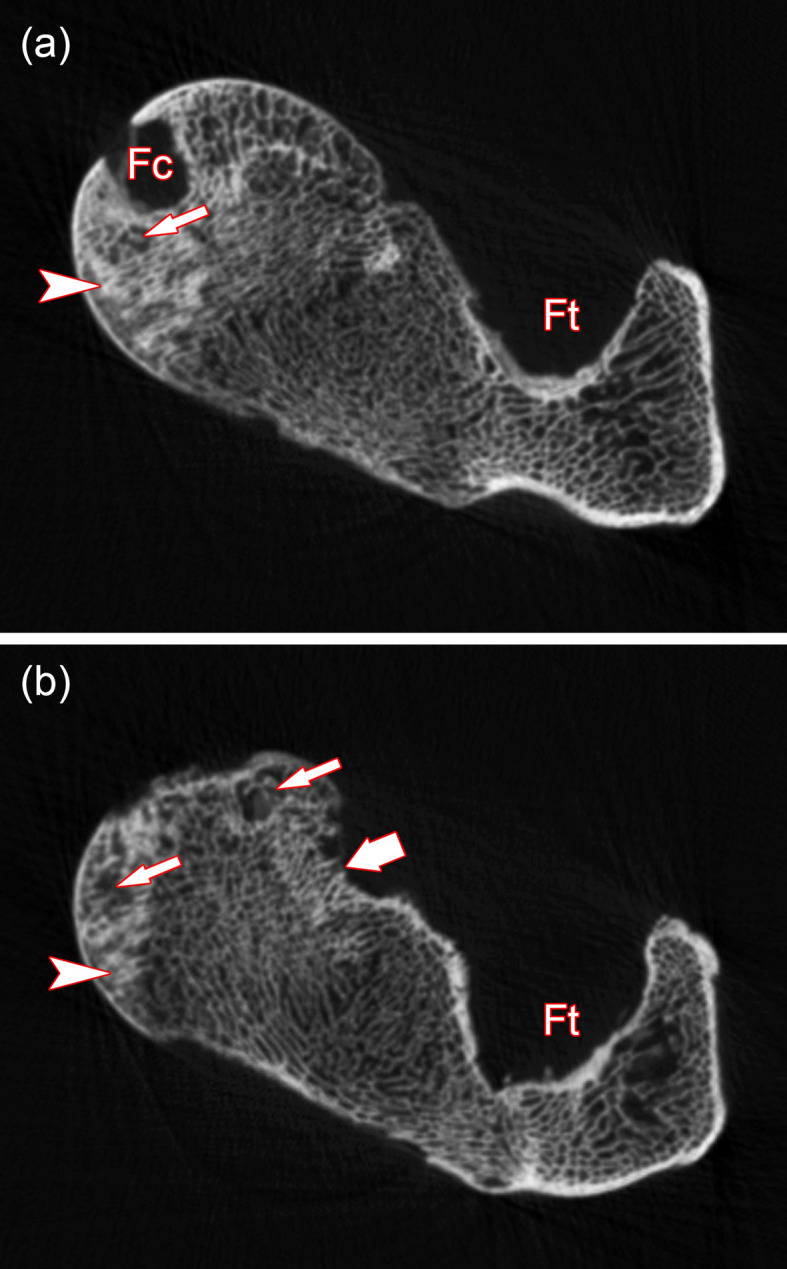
CT-images (transverse slices) of the proximal portion (femoral head region) of the right pathological femur of the roe buck. (a) Slice located in the region of the fovea capitis ossis femoris (Fc). (b) Slice located further distally. Ft: fossa trochanterica, arrowheads: areas of bone sclerosis, thin arrows: lucent areas indicative of bone resorption, thick arrow: bone defect.
